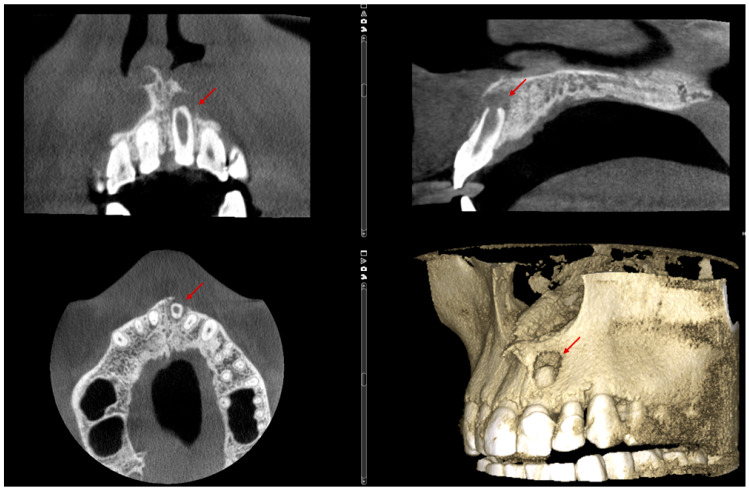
The labial bone fenestration is visible on the CBCT image (indicated by red arrows).
